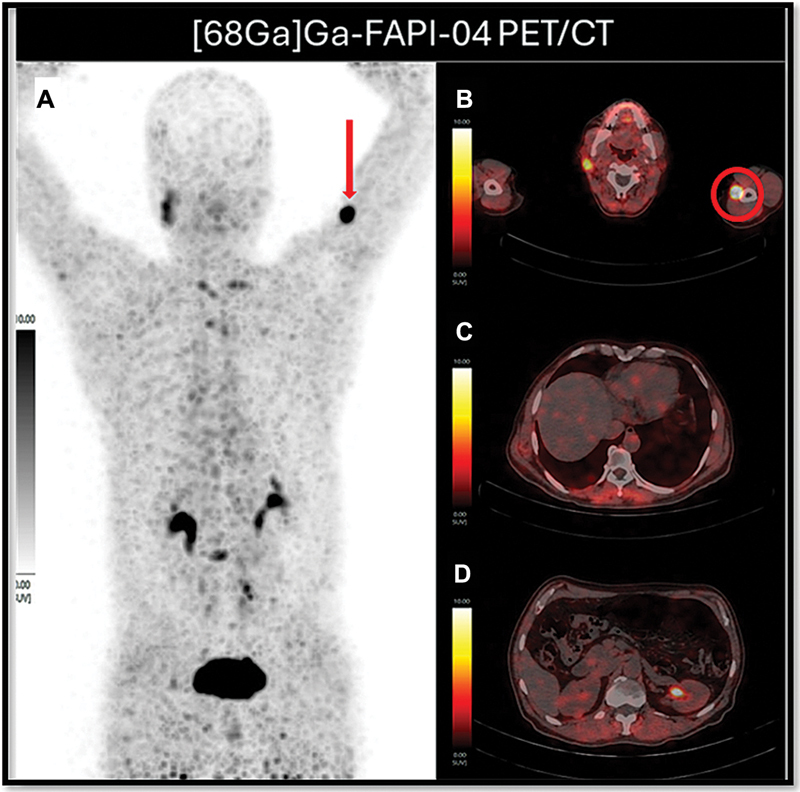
MIP of [68Ga]-FAPI-04 PET/CT (
